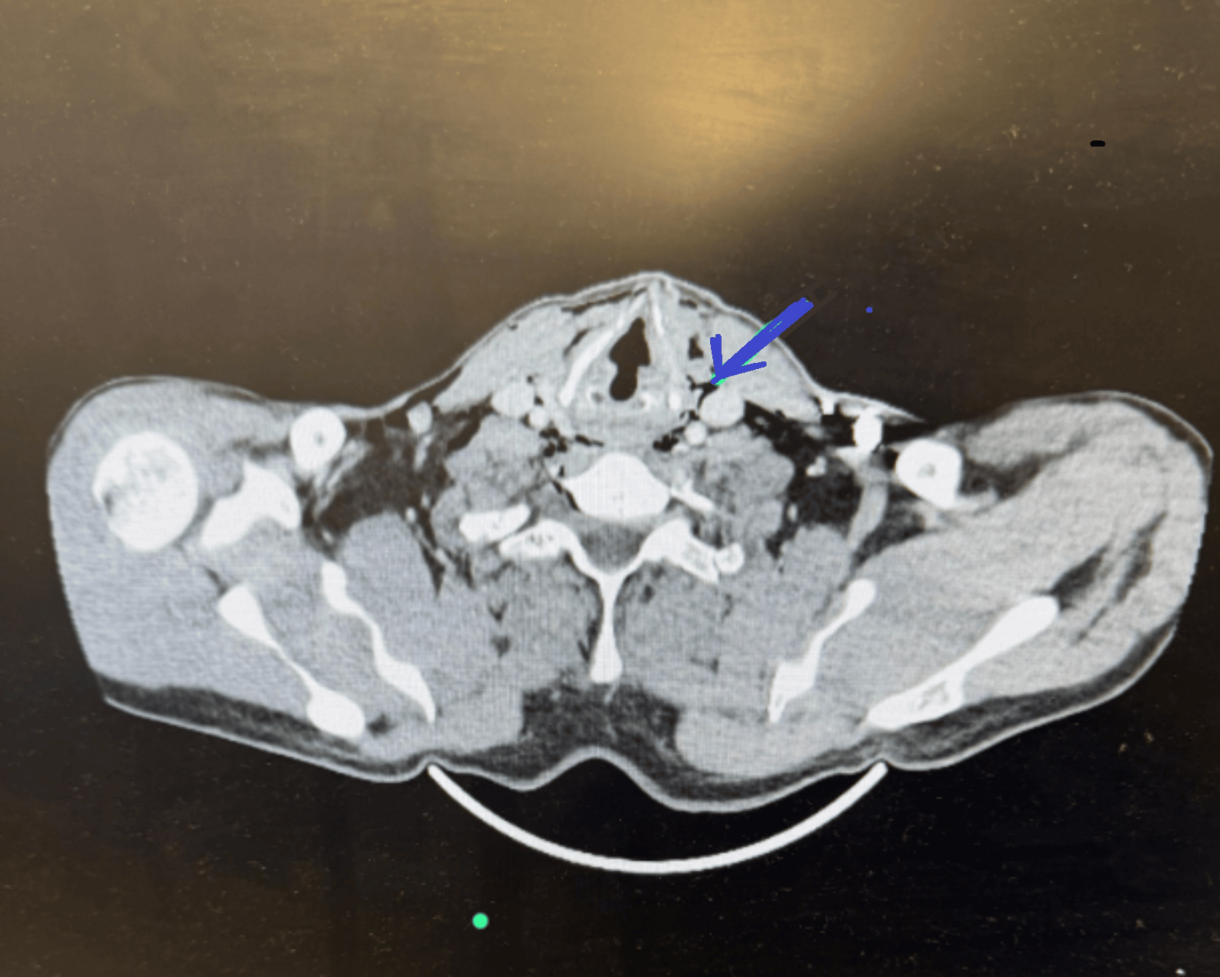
CT of the neck with contrast showing emphysema due to rupture
